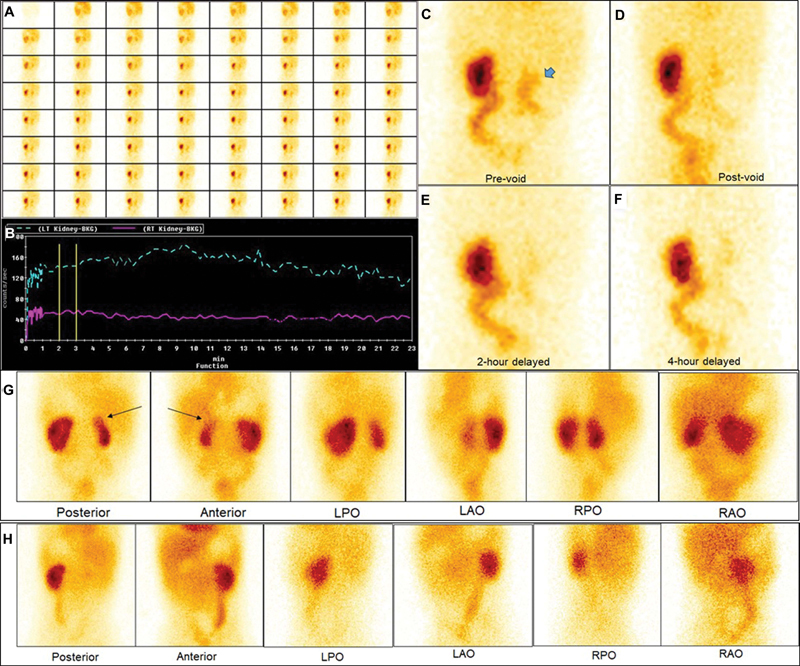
A 10-month-old girl with high ARM syndrome, recto-vestibular fistula, bilateral ectopic ureters, and left megaureter. She underwent anoplasty, colostomy with fistula repair, left ureteric reimplantation, and ureterostomy. The Tc-99m EC scintigraphy (
